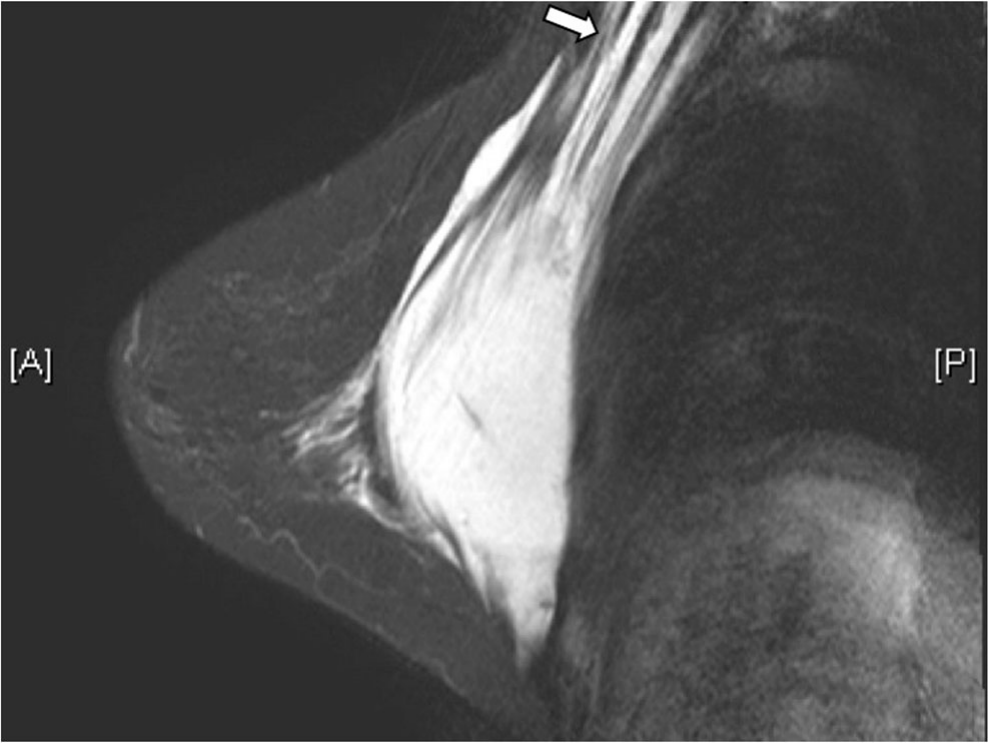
Intrapectoral migration of PAAG. T2-weighted, fat-saturated sagittal MR image reveals PAAG tracking along fascial planes of the pectoral muscle (arrow)
